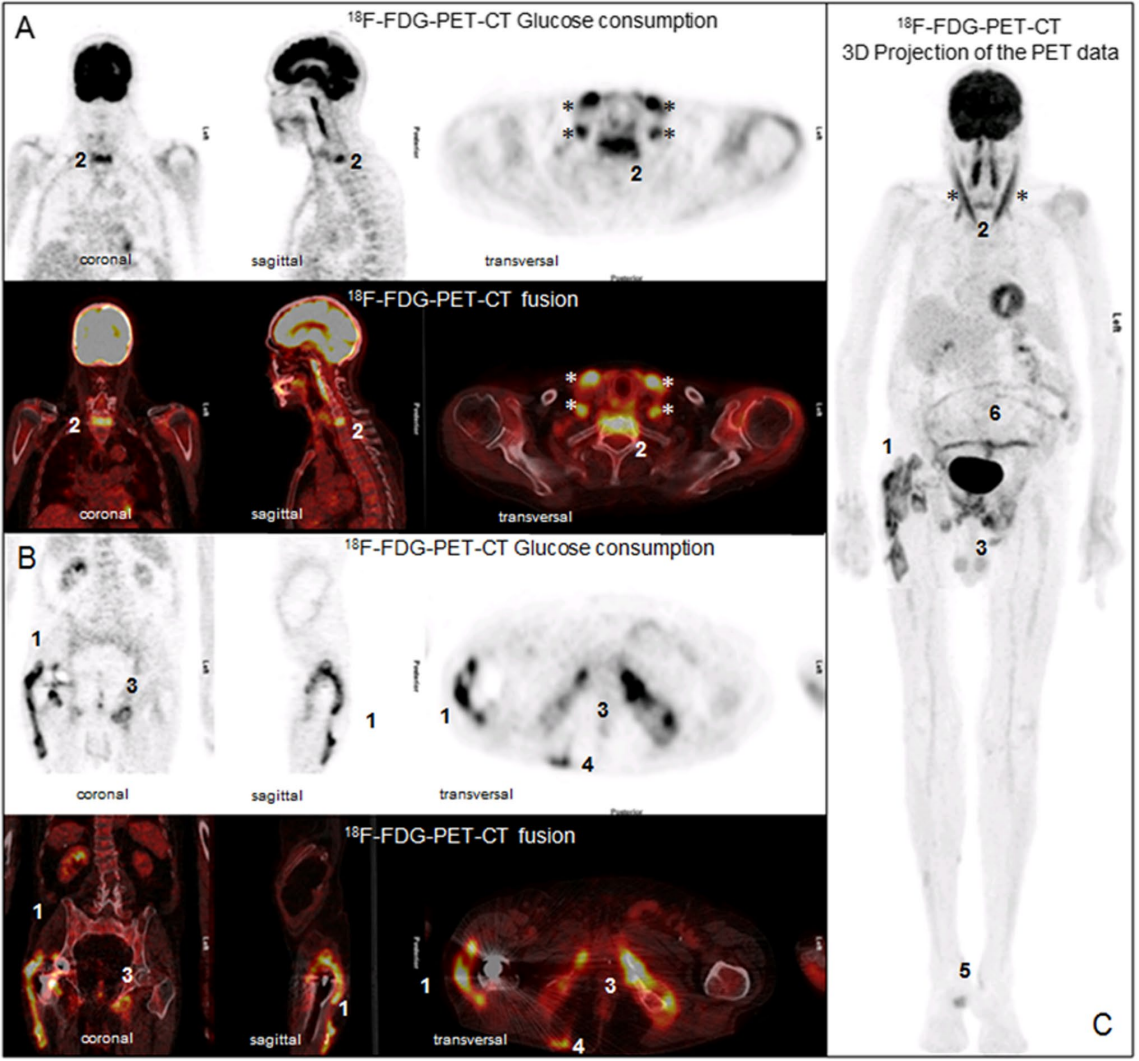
Representative PET-CT images of A head and thorax, B abdomen and pelvis in coronal, sagittal, and transversal orientation. Panel C shows a 3 D projection of the ^18^F-FDG-PET data. (1) Patient with infection in the right total hip arthroplasty with intensive ^18^F-FDG uptake in the periprosthetic soft tissues next the acetabulum, on both sides of the neck of the prosthesis and along the whole lateral part of the femoral component with also extensive infiltration into the surrounding periprostetic soft tissues. Additional ^18^F-FDG-PET-positive findings: (2) spondylodiscitis in C7/ Th1; (3) fracture of the left os oschii, left and right os pubis; infectious focus in the surrounding soft tissues; (4) fistula along the right M. gluteus maximus to the skin surface; (5) soft tissue infection at right calcaneaus; (6) enteritis of the small intestine. (*) Physiologic ^18^F-FDG uptake in neck muscules
